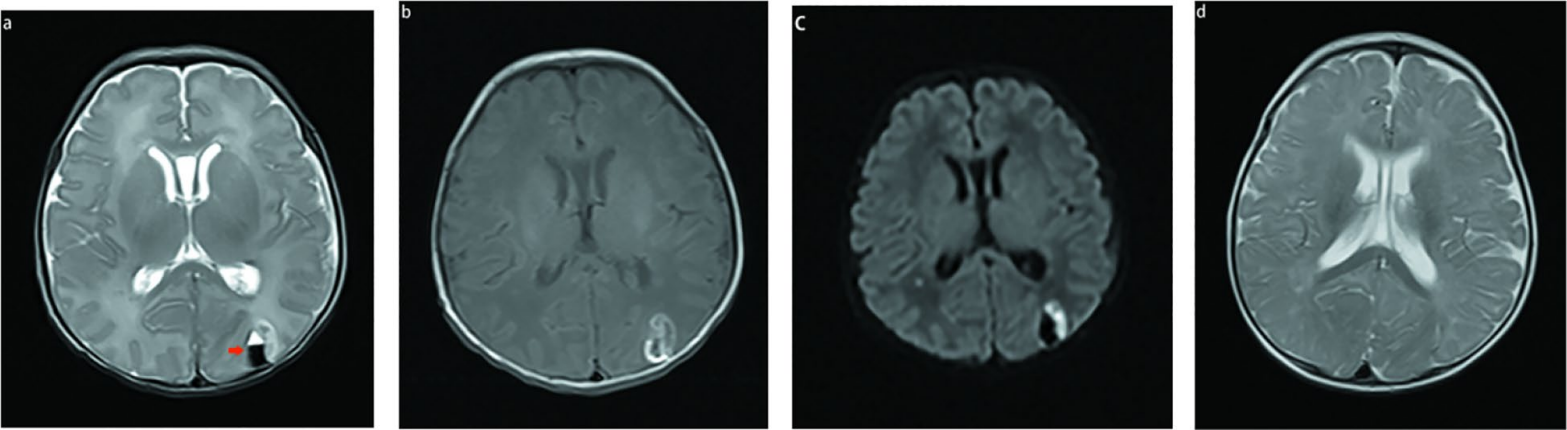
MR image of a full-term neonates with pattern B. The first MRI was performed at 5 days after birth (a–c). The subpial hemorrhage shows hypointense on T2WI and adjacent cortical infarction shows hyperintense (a), we found fluid–fluid level on subpial hemorrhage(arrow). Mixed signal on T1WI (b). There was no restricted diffusion in subpial bleed and hyperintense on cortical infarction (c). This combination of a dark subpial hemorrhage collection and the bright underlying cerebral cortex forms yin yang sign (a and c). Reexamination after 8 months showed that the subpleural hemorrhage had been basically absorbed, and there was no cortical infarction signal (d).
